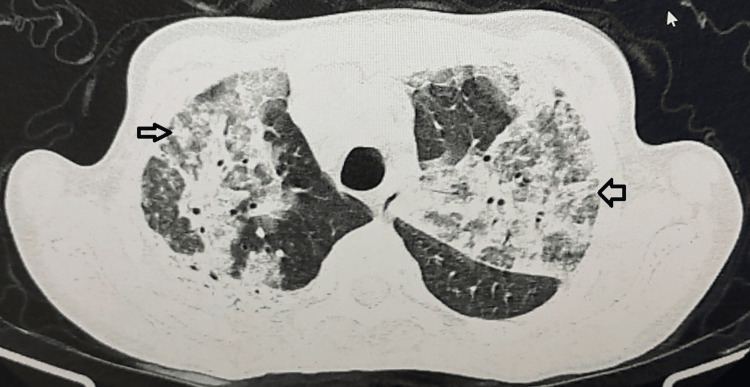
Case 2: Axial chest CT scan in the parenchymal window showing bilateral areas of consolidation associated with diffuse bronchial dilatations (bronchiectasis) in a patient with common variable immunodeficiency.
